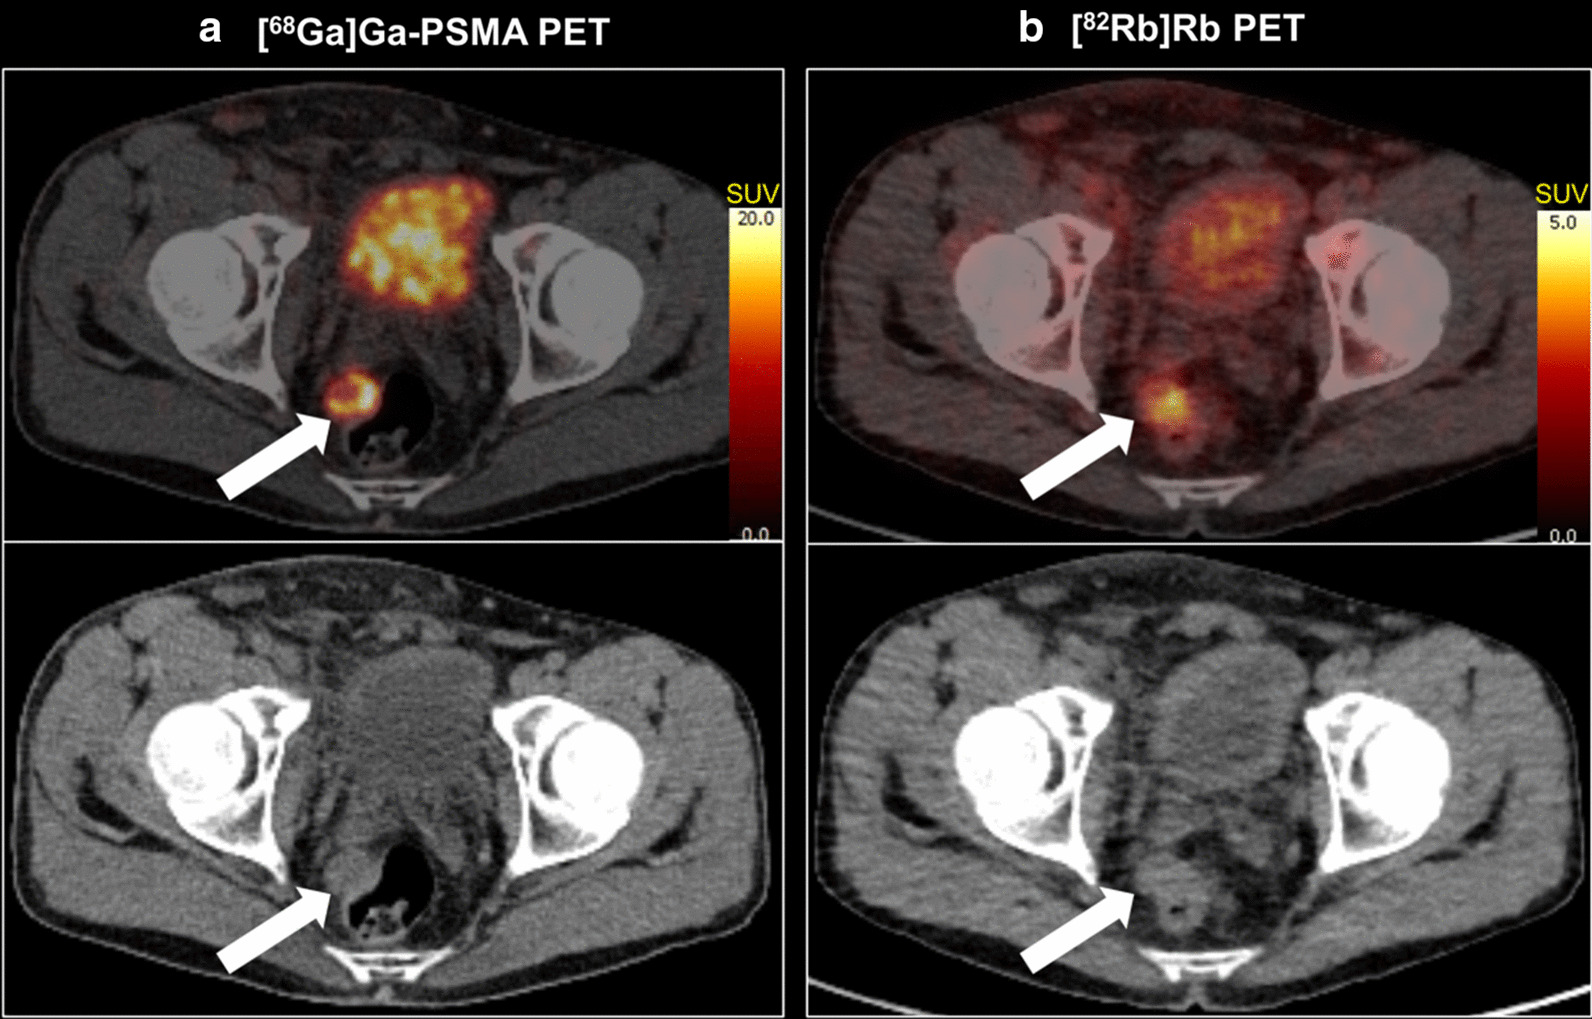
Large pararectal lymph node metastasis with both highly increased [^68^Ga]Ga-PSMA-11 uptake (a) and [^82^Rb]Rb uptake (b) on transaxial fused PET/CT images
